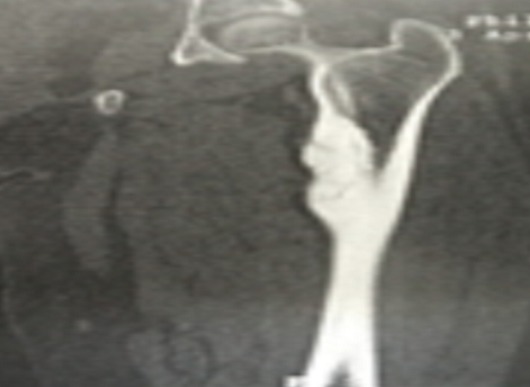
The caracteristic candle wax condensation of the proximal femur below the calcar in the CT scan
